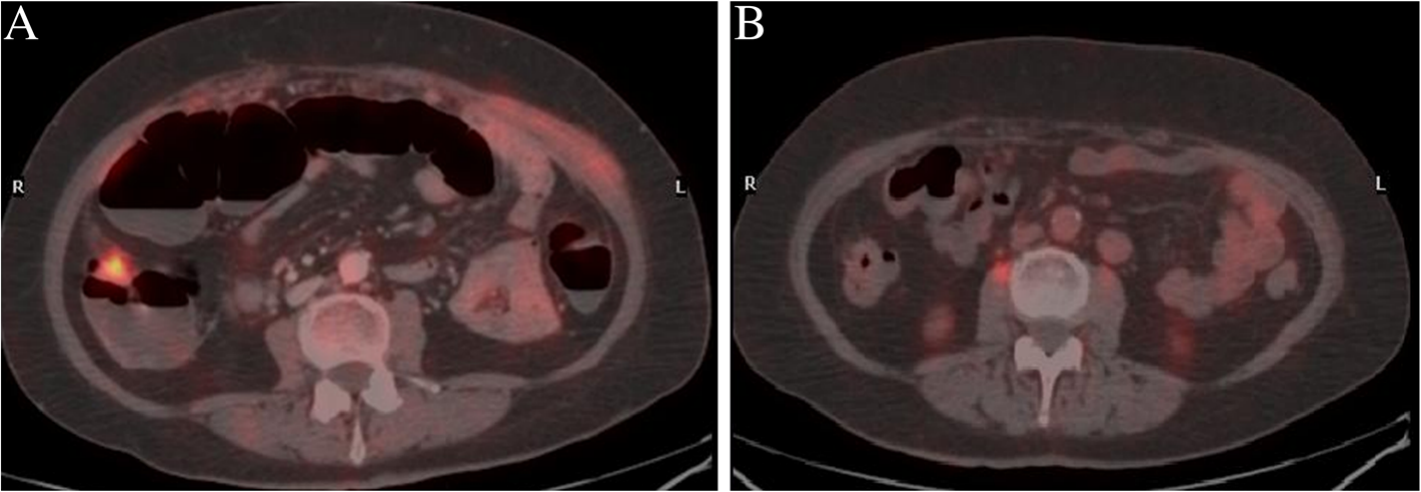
Peritoneal carcinomatosis from ovarian cancer detected by^18^ F-FDG PET/Contrast enhanced (Ce)CT before starting bevacizumab (A) and at best response (B).
